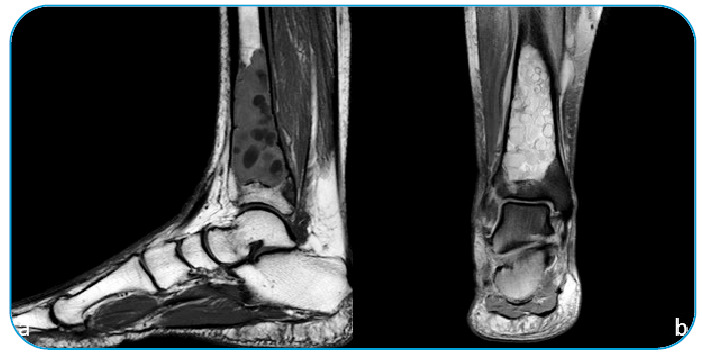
A sagittal (a) and coronal (b) slice of the MRI
